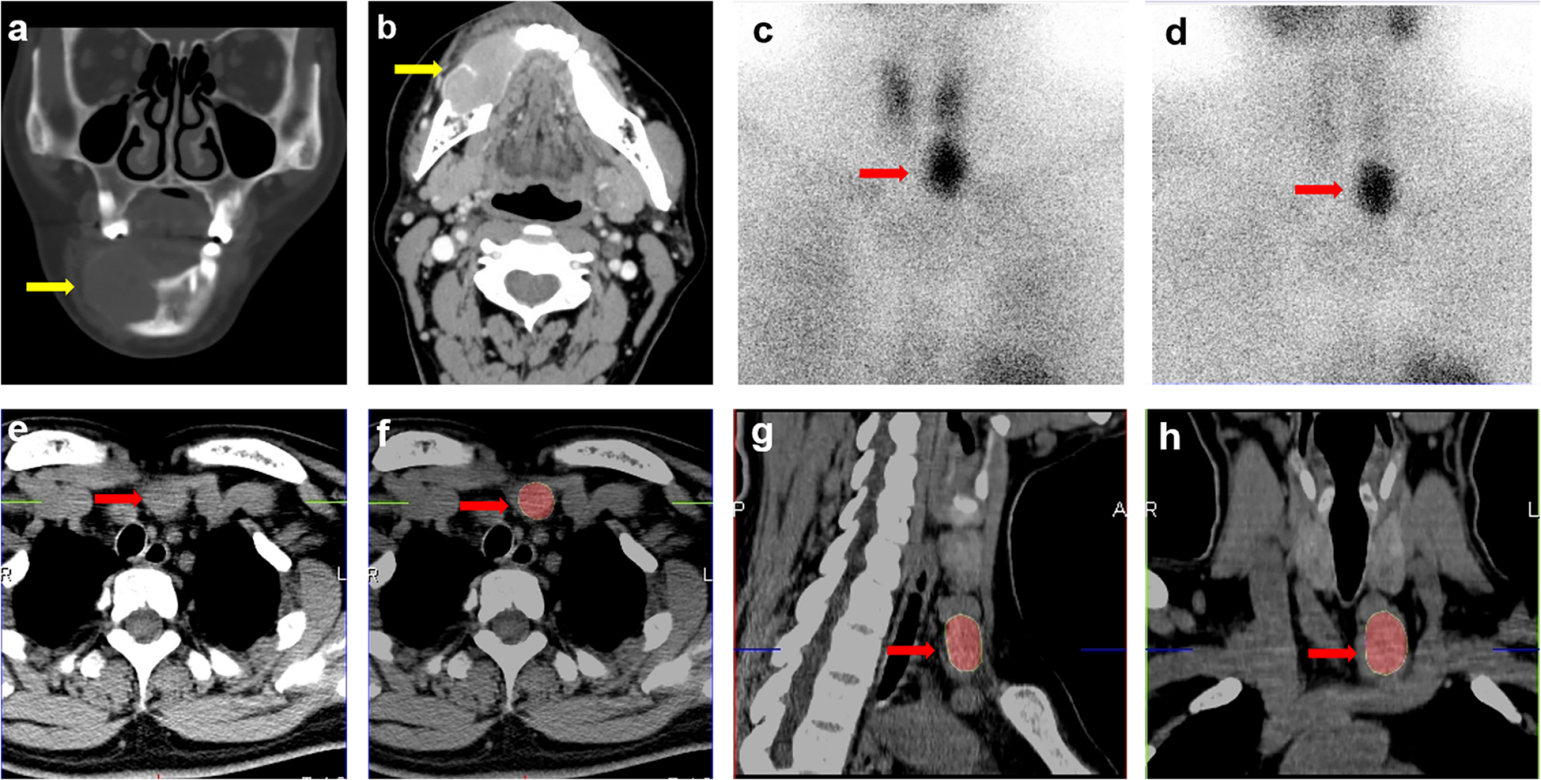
(a-b) Enhanced computer tomography scan of the brown tumor in the mandible (yellow arrows). (a) Coronal CT and (b) axial CT. (c-d) ^99m^Tc-MIBI of the parathyroid adenoma (red arrows): the early imaging (c) shows the radioactive concentration at the site of the left parathyroid, and the delayed imaging (d) shows that it did not degrade. (e-h) SPECT/CT scintigraphy revealed the low-density lesion with high radioactive uptake at the site of the left parathyroid (red arrows): (e-f) Axial, (g) sagittal, and (h) coronal images of the parathyroid adenoma
